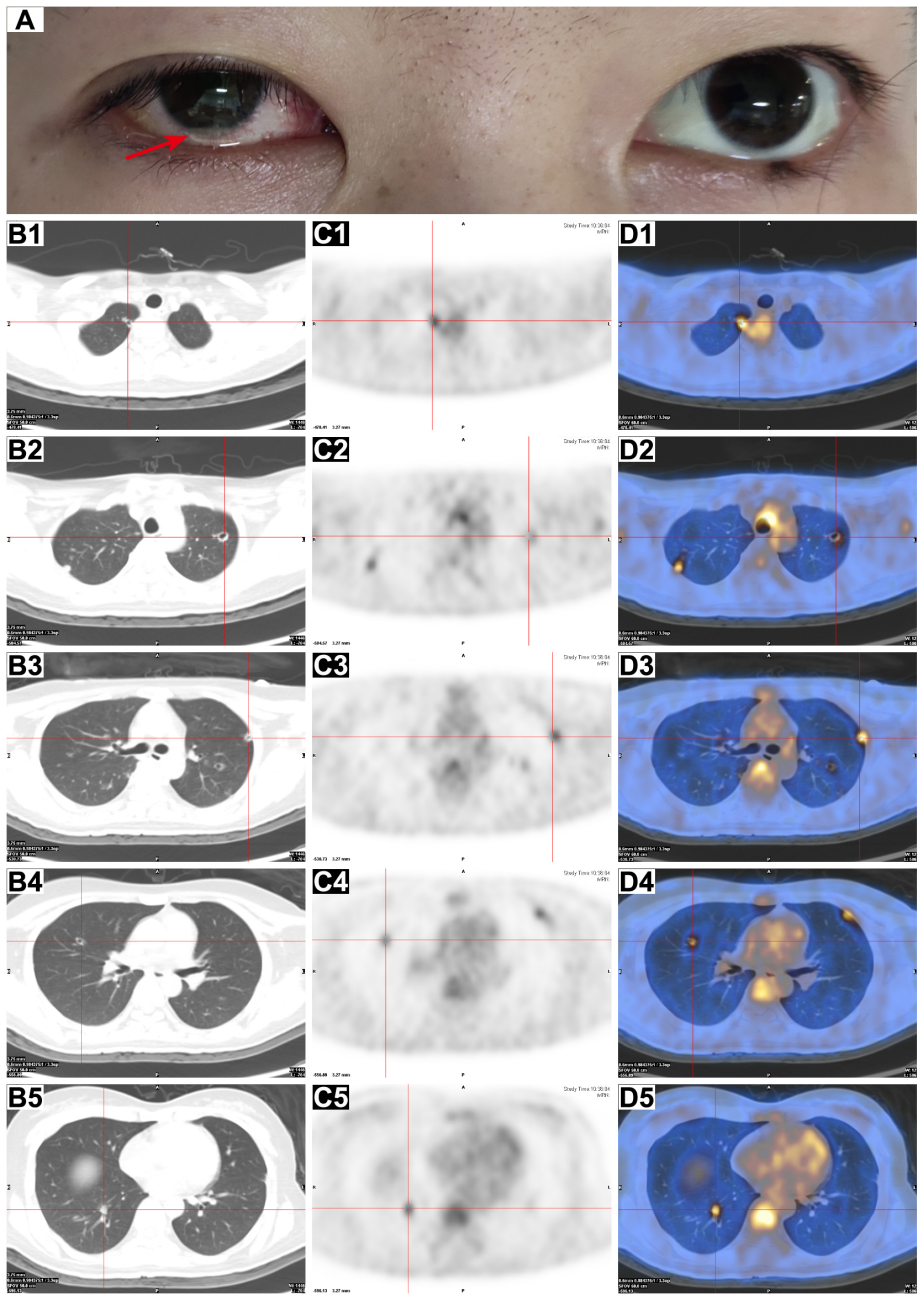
Ocular appearance and PET/CT scan of the chest. (A) Clinical photograph of the patient on day 13 after admission, showing conjunctival congestion in the right eye with pus in the anterior chamber (red arrow). (B–D) Axial chest CT (B1–B5), PET (C1–C5), and fusion PET/CT (D1–D5) images showed the presence of multiple cavitary nodules in both lungs with increased FDG uptake (red crosses).
